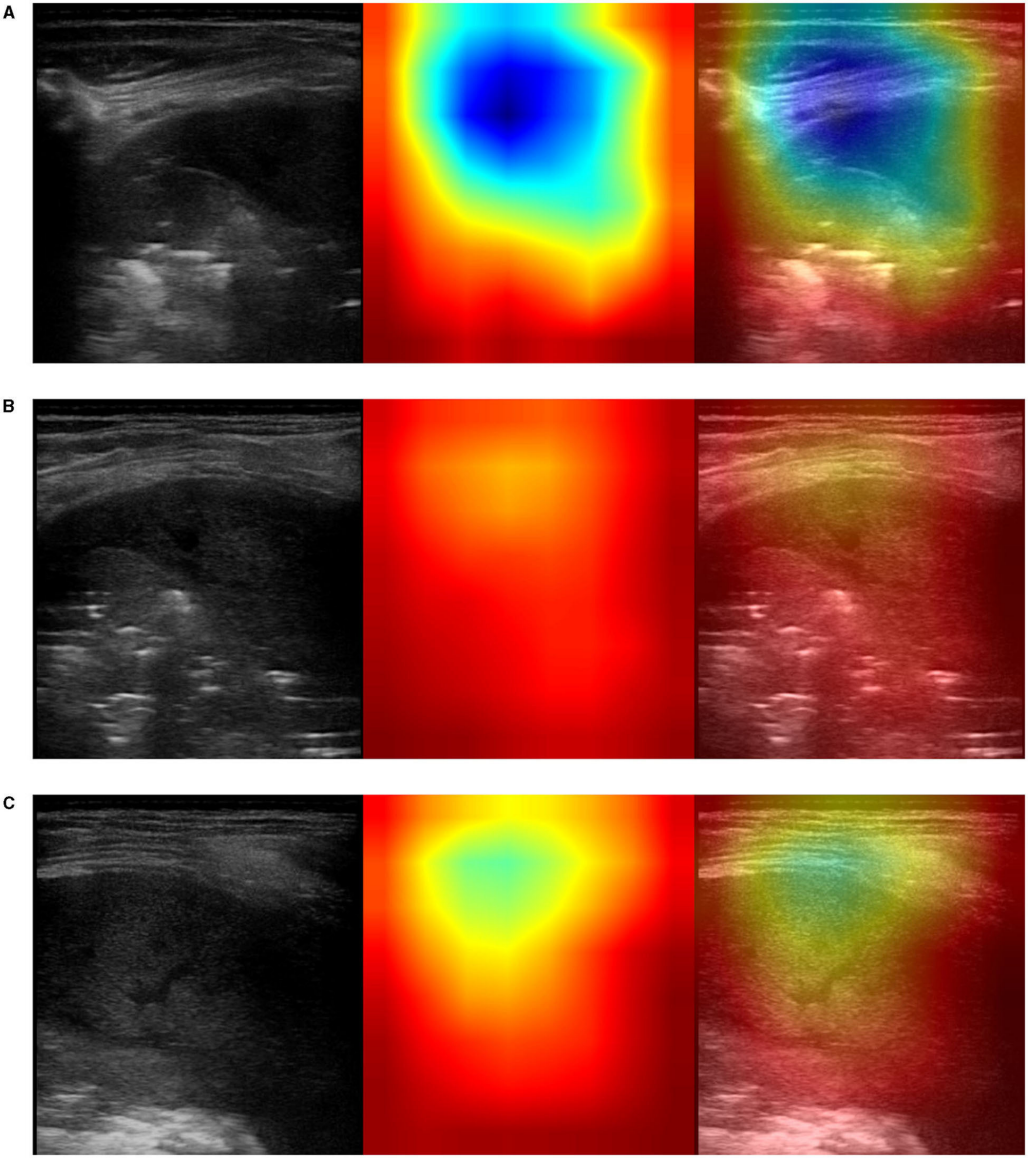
Example of lung ultrasound images correctly predicted by the best performance model (Inception-v3) as bacterial pneumonia. Left: original LUS image; middle: Grad-CAM visualization; right: the class activation mapping transparently overlaid on the original LUS image. Note that red and orange regions correspond to high scores for the predicted class and correctly highlight diagnostic-relevant features: (A) severe consolidations with fluid bronchogram and dynamic air bronchogram, (B) severe consolidations with fluid bronchogram and dynamic air bronchogram, complicated pleural effusions, (C) fibrinous pleural effusions with pulmonary atelectasis.
